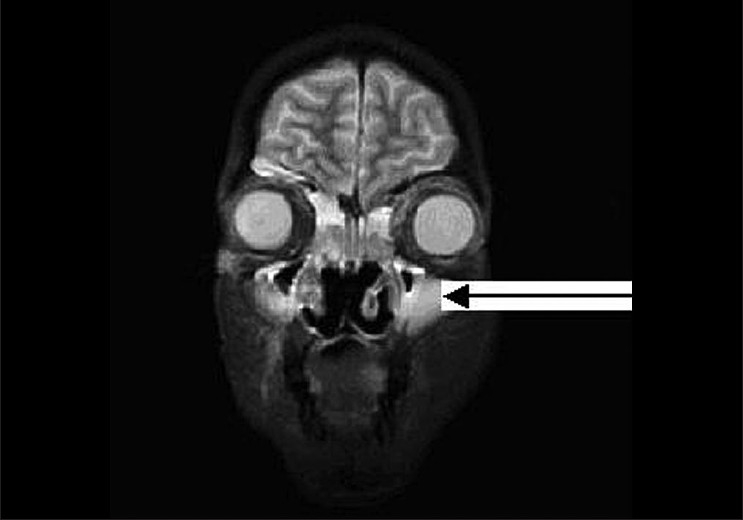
MRI scan showing swollen mucosa in the bilateral maxillary sinus with fluid collection (arrow)
